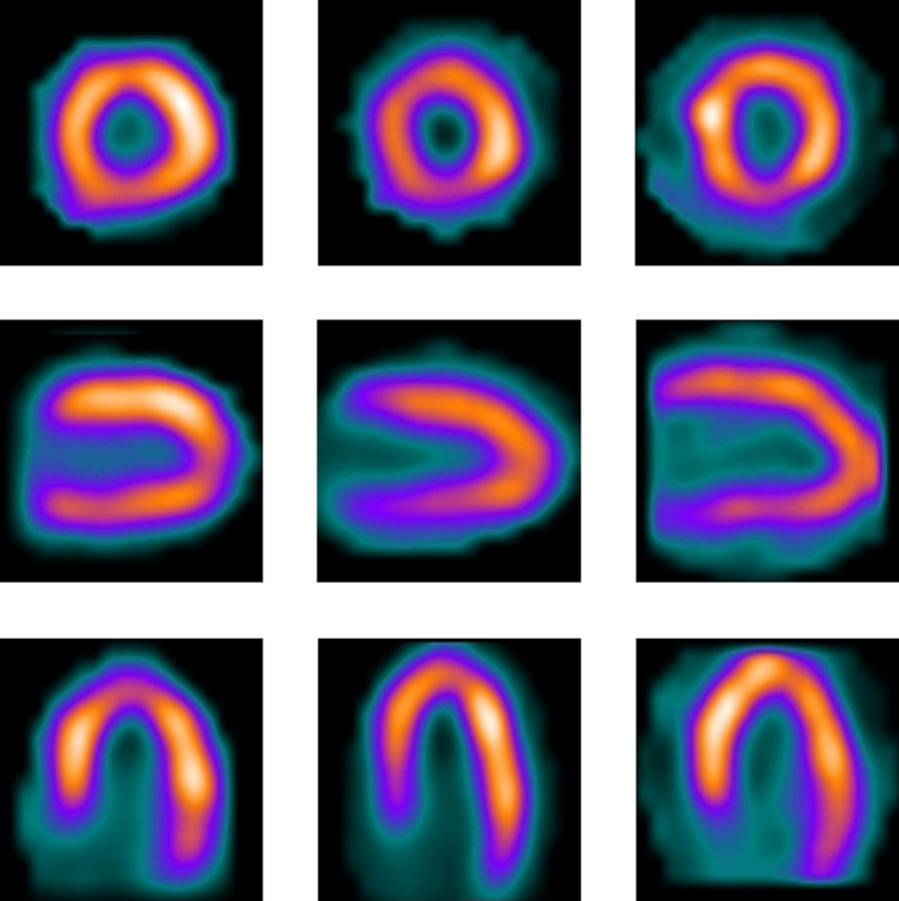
Example of constant image quality in MPI SPECT scans of three male patients without any perfusion defects with varying body weights. From left to right: 66 kg (22.6 kg·m^−2^), 85 kg (25.1 kg·m^−2^), and 124 kg (34.0 kg·m^−2^). The corresponding *short*, *vertical long* and *horizontal long axes* are shown from *top* to *bottom*. A patient-specific tracer activity was applied (330, 395, and 555 MBq, respectively), using a fixed scan time. The image quality of all three sets was scored as ‘good,’ independent of patients’ size
